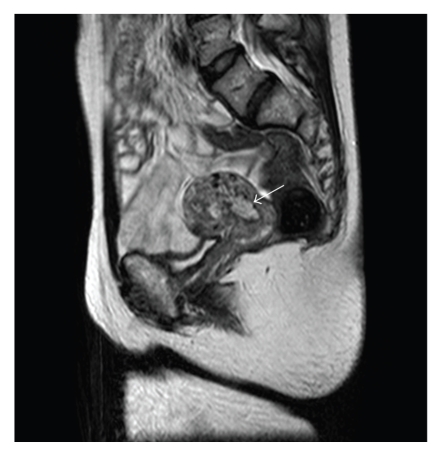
MRI at 47 days after delivery. Arrows point to placental polyp.
